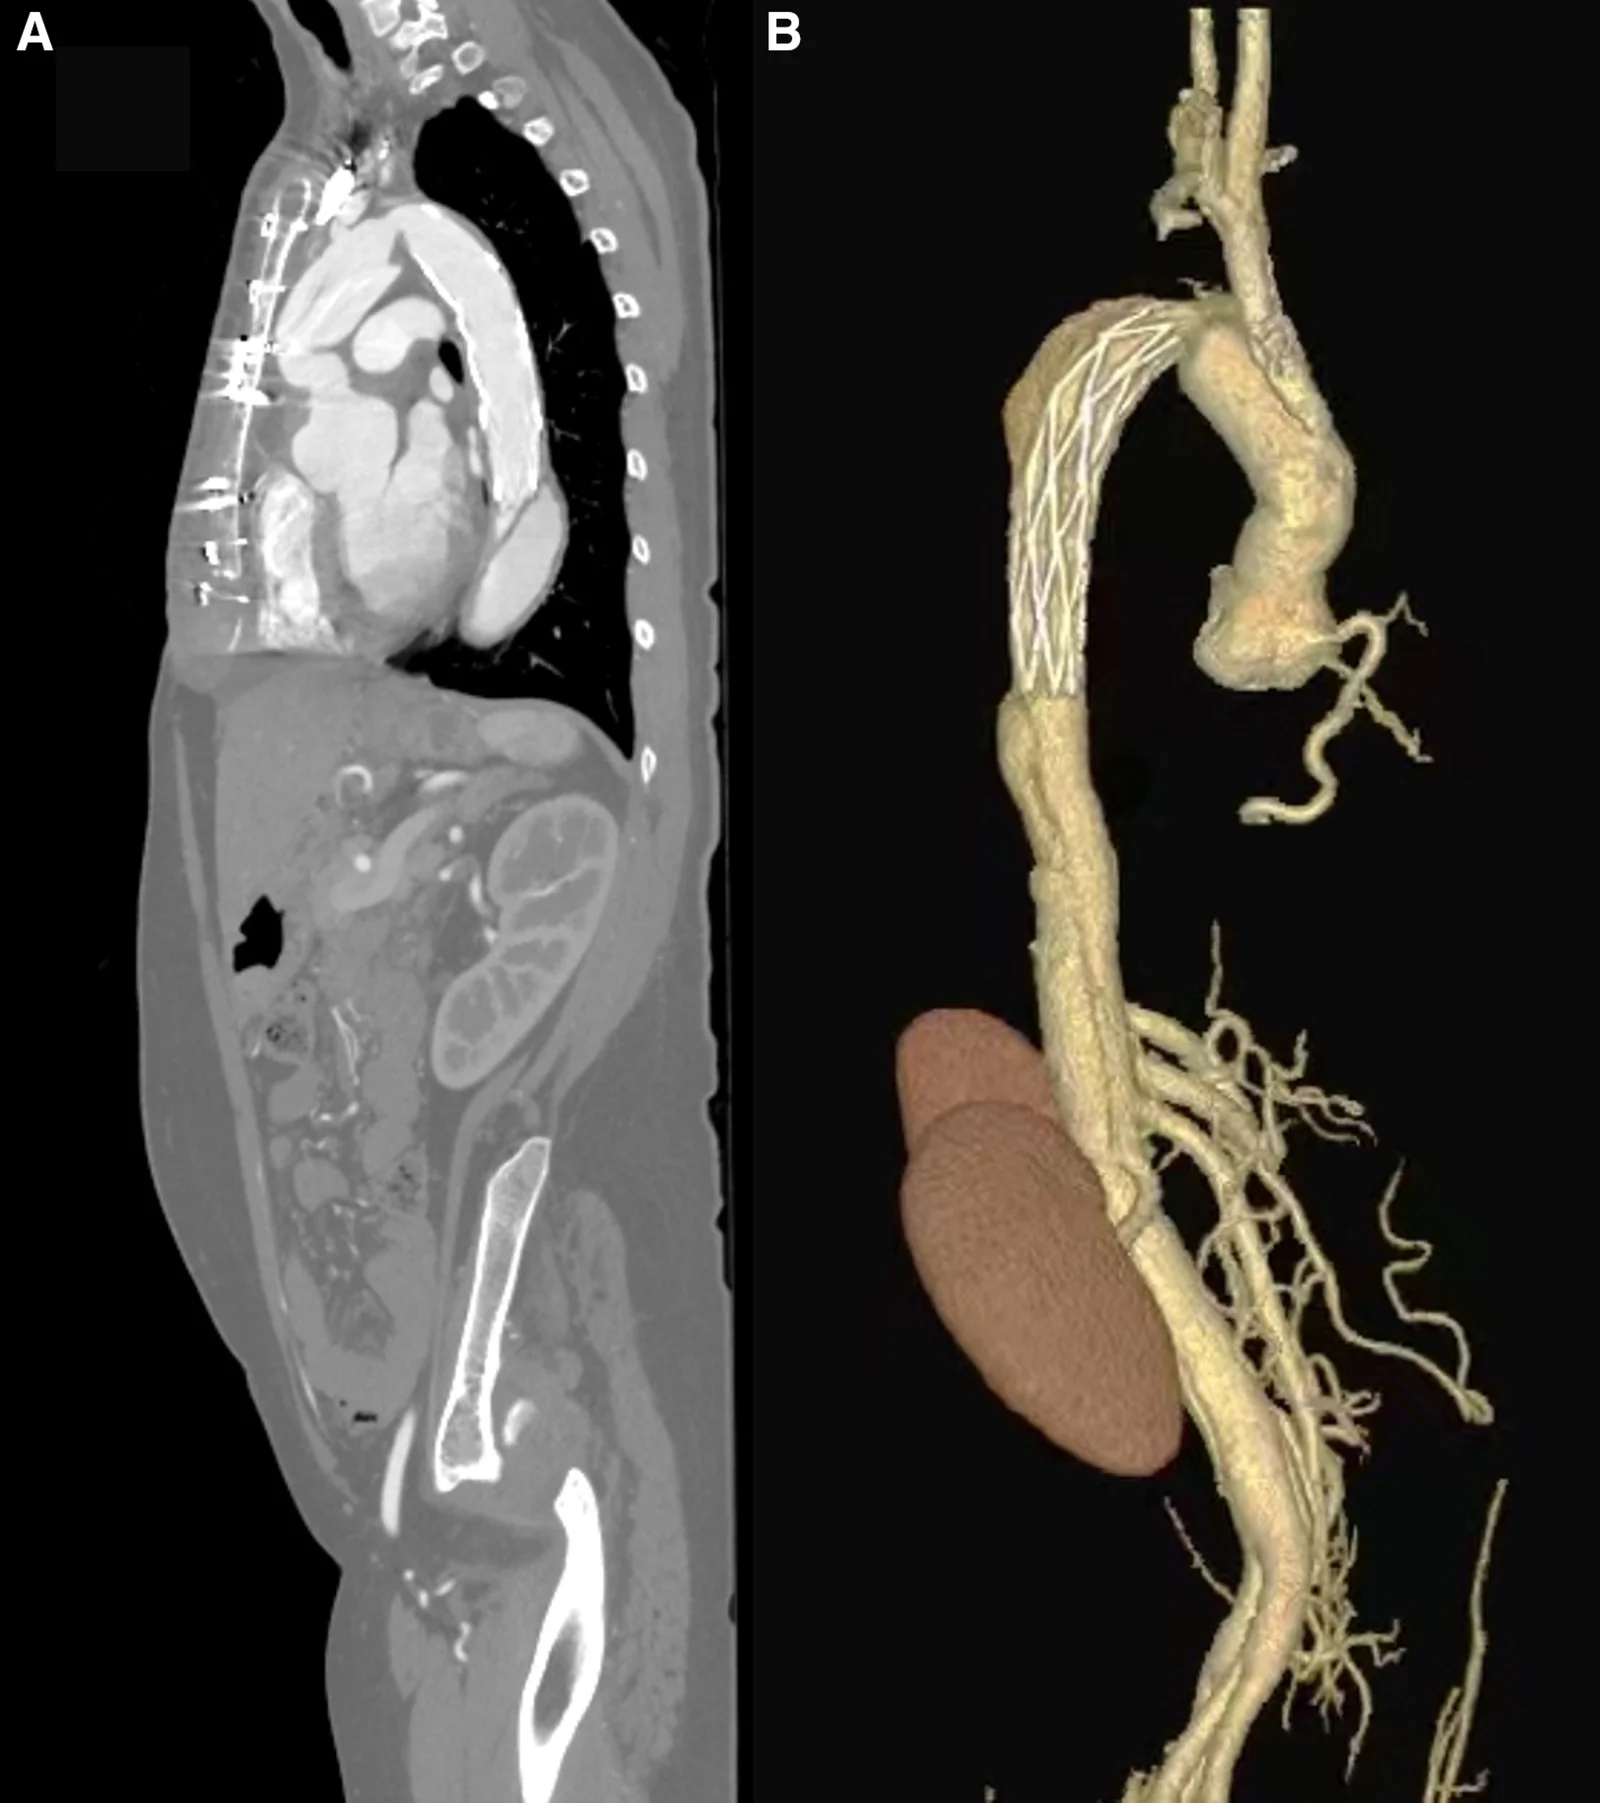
Postoperative computed tomography angiography (CTA) at 1-month follow-up. (A) CTA image obtained 1 month postoperatively, showing intact anastomoses without any signs of endoleak or pseudoaneurysm. (B) Three-dimensional (3D) reconstruction of the aorta confirming excellent patency of the graft and the reconstructed arch vessels.
